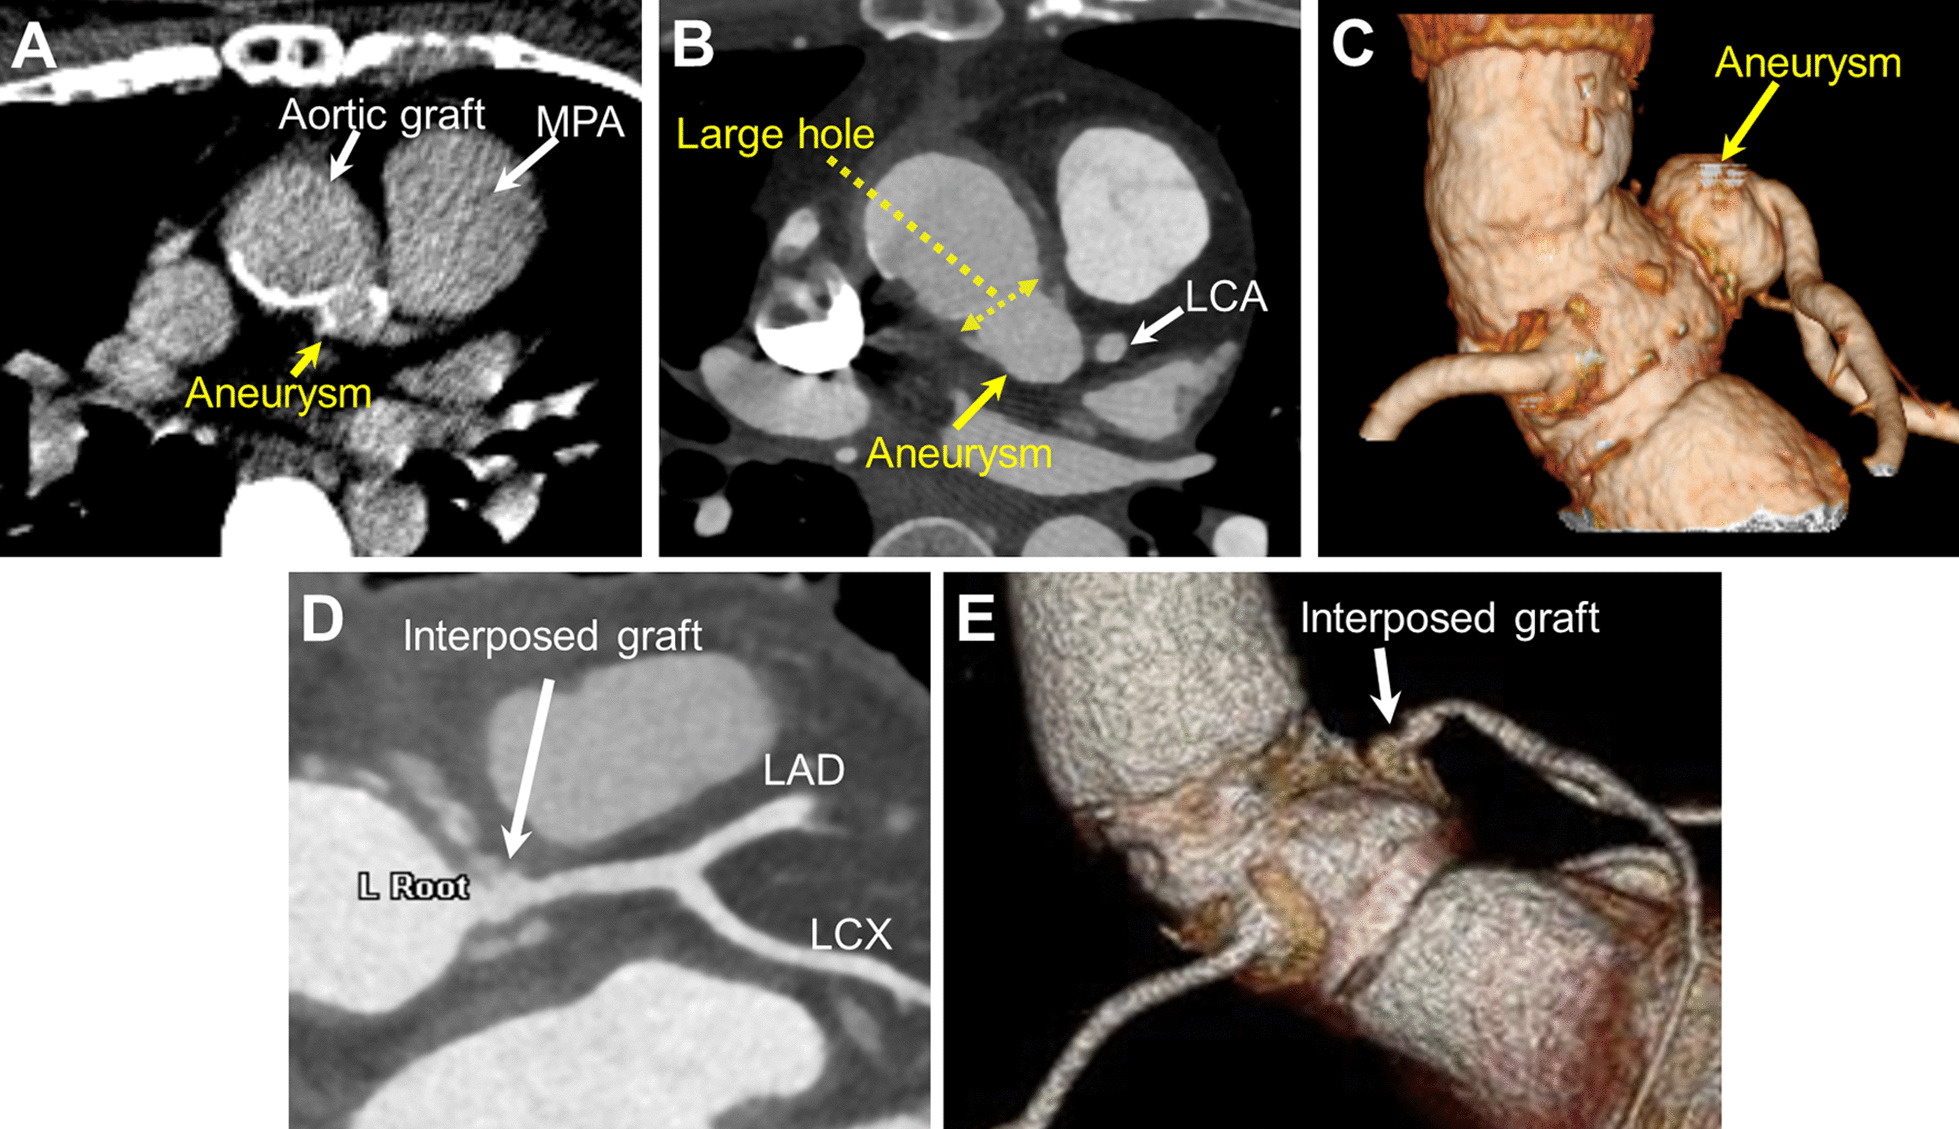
A Computed tomographic (CT) image 14 years after aortic root replacement shows the LCA button aneurysm with a diameter of 10 mm. B CT image 24 years after aortic root replacement shows that the LCA button aneurysm gradually enlarged to a diameter of 20 mm. C Preoperative three-dimensional reconstructed CT image shows the LCA button aneurysm. D, E Postoperative CT image shows successful reconstruction of the LCA system. LAD: left anterior descending artery, *LCA* left coronary artery, *LCX* left circumflex artery, *MPA* main pulmonary artery
